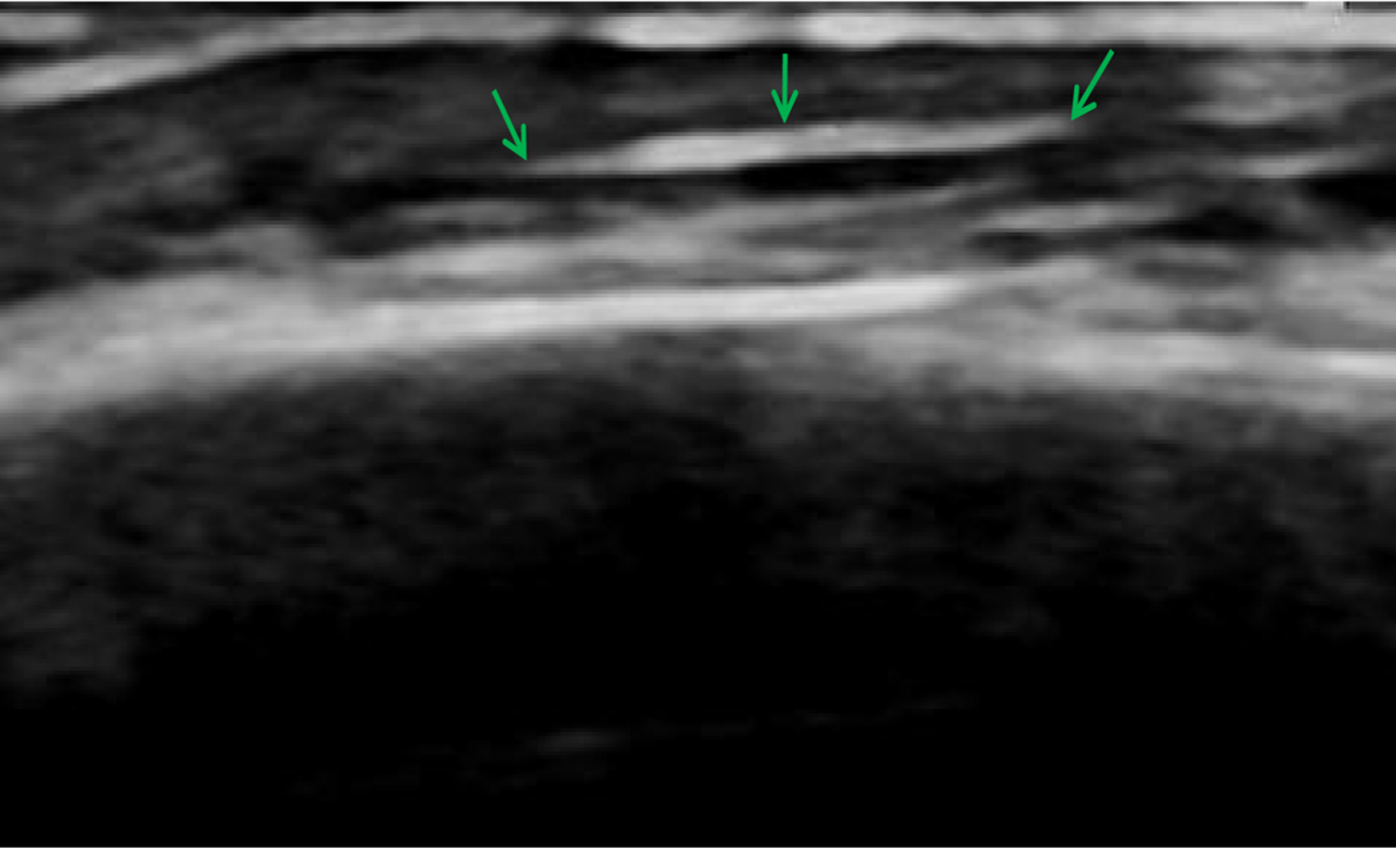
B-ultrasound confirmed that the subcutaneous indwelling needle showed a thin strip of echo under the scalp.
